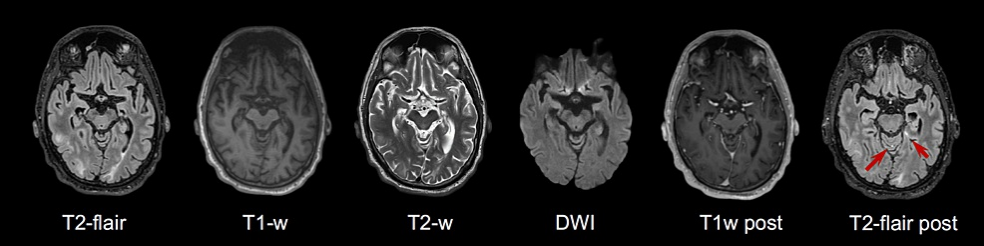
Representative pathological changes on various sequences on MRI of the brain, day 16 Hyperintensities in subcortical and juxtacortical white matter on T2-FLAIR and T2-W with corresponding hypo-intensities on native T1-W image. No diffusion restriction was detected on DWI and ADC maps, and no pathological contrast enhancement was found on postcontrast T1-W image (T1-W post), minimal punctate patchy leptomeningeal enhancement was detected on postcontrast T2-FLAIR images (indicated by arrows).
